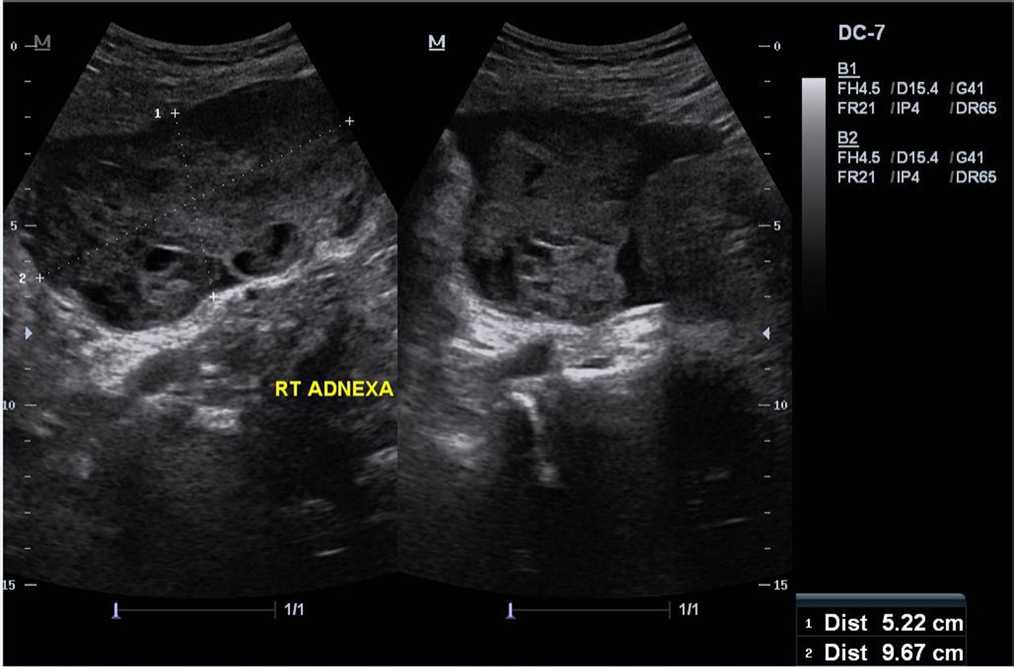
Pelvic ultrasound images demonstrate a heterogeneous cystic mass with solid components measuring 5 × 9 cm in the right adnexa. There is associated ascites noted.
